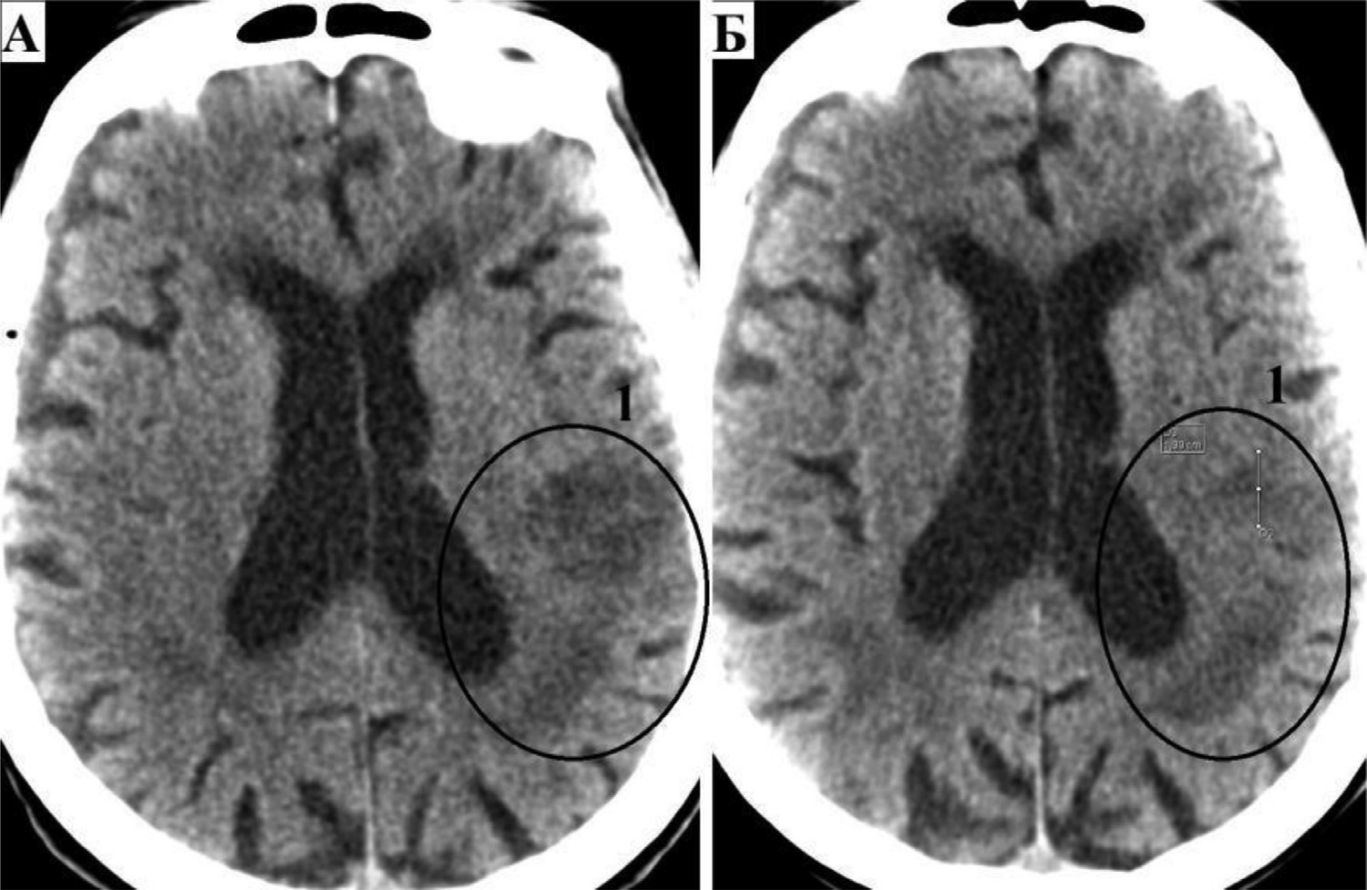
Computed tomography of the brain. (A) Computed tomography of the brain upon admission to the hospital: 1 - ischemic stroke in the basin of the left middle cerebral artery. (B) Computed tomography of the brain on day 22 after surgery: 1 - decrease in perifocal edema and the volume of the ischemic focus in the basin of the left middle cerebral artery.
